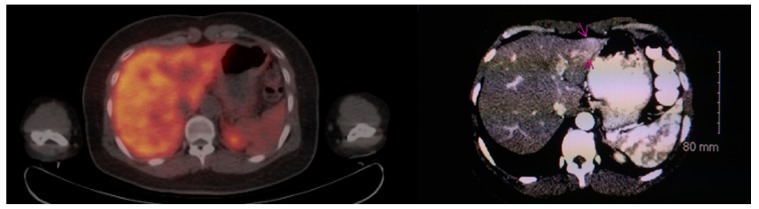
Primary HCC demonstrating iso-intense FCh uptake in non-cirrhotic patient. PET/CT image (left) shows no focal area of increased FCh uptake in the liver. However, a diagnostic CT scan with intravenous contrast (right) revealed a mass showing arterial enhancement and delayed washout in the left hepatic lobe lateral segment (*purple arrows*). Biopsy of this mass confirmed it to be a well-differentiated HCC.
